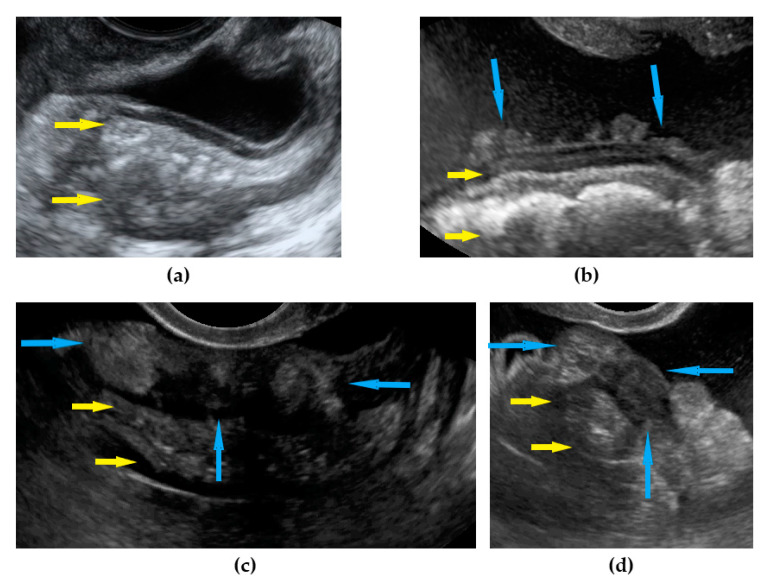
Ultrasound images of rectum: (a) normal rectum; (b) nodular carcinomatosis on the peritoneal surface of the rectum; (c) sheet-like involvement of the rectum wall (longitudinal view); (d) sheet-like involvement of the rectum wall (transverse view). Yellow arrows identify rectum, blue arrows identify lesions.
